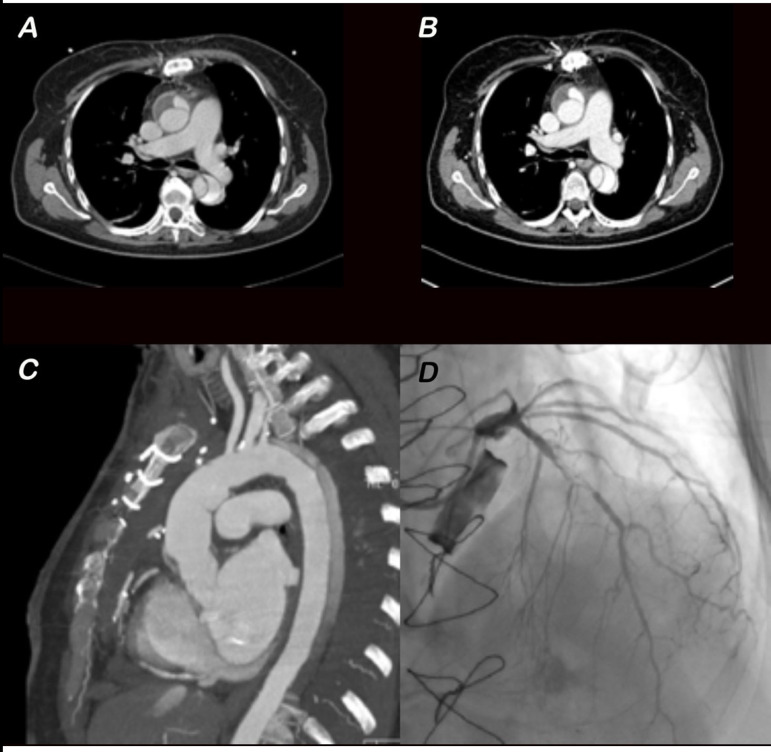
A) and B) Computed tomography imaging of iatrogenic acute aortic dissection in 7th and 19th postoperative days revealing persisting type A aortic dissection with false lumen without thrombosis in ascending aorta. C) Computed tomography performed after ascending aorta and hemiarch replacement. D) Angiography showing spontaneous dissection of left anterior descending coronary artery.
